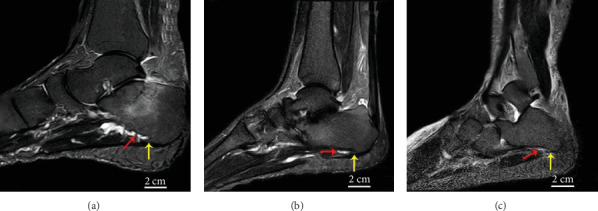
Feet MRI of three patients. The red arrows point to the plantar fasciitis, and the yellow arrows point to the plantar calcaneal spur.
